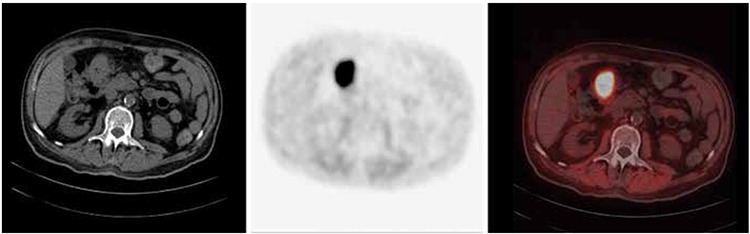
A 57-year-old-male patient with a diagnosis of metastatic adenocarcinoma on liver biopsy with unknown primary. Axial positron emission tomography-computed tomography and fusion images demonstrate hypermetabolic thickening of the transverse colon (SUVmax: 14.6). Biopsy of the transverse colon revealed adenocarcinoma
